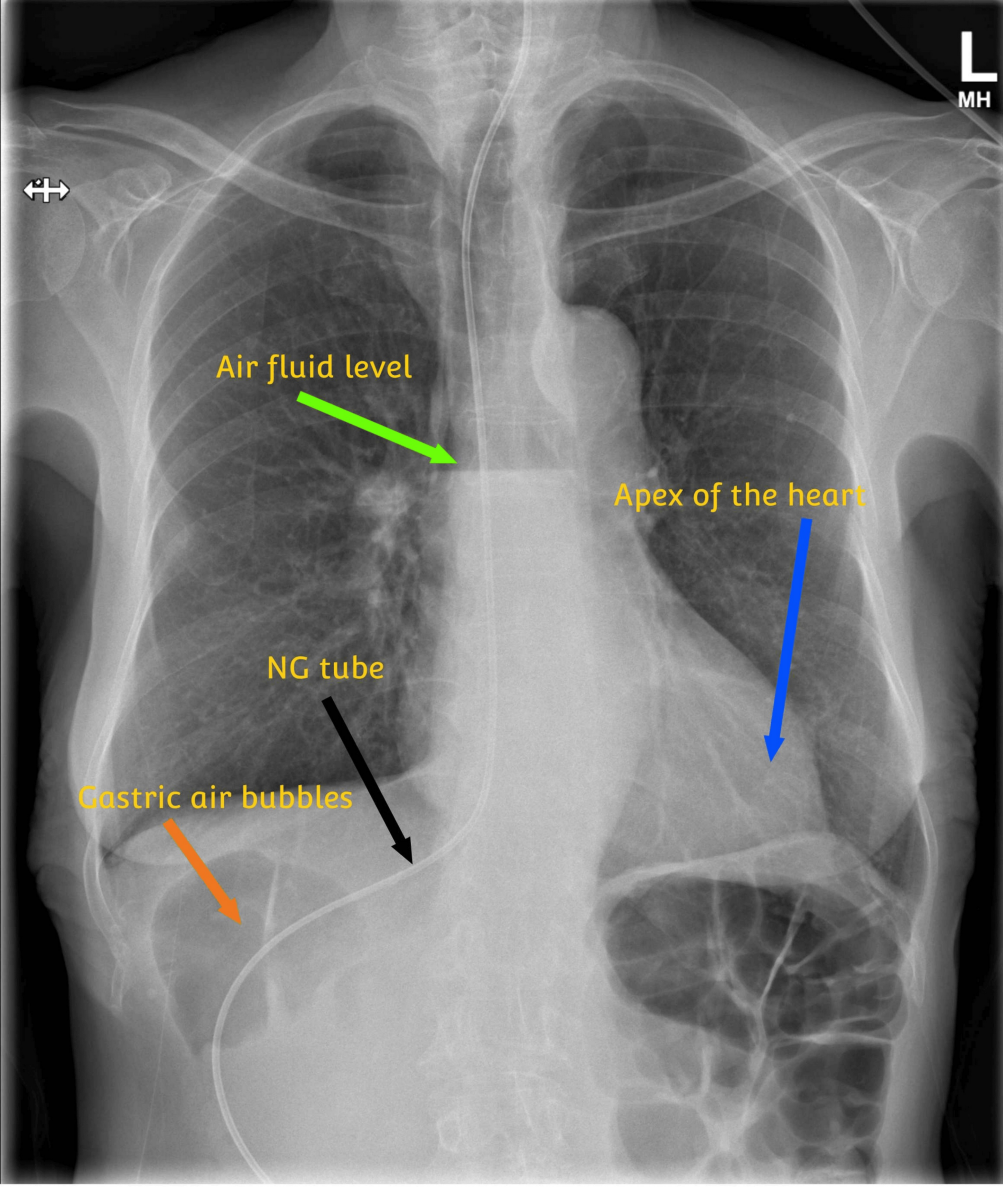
Posterior-anterior view chest X-ray showing the apex of the heart on the left side, nasogastric tube curving to the right below the diaphragm, gastric air bubbles on the right side, and an air-fluid level due to esophageal obstruction NG: nasogastric
